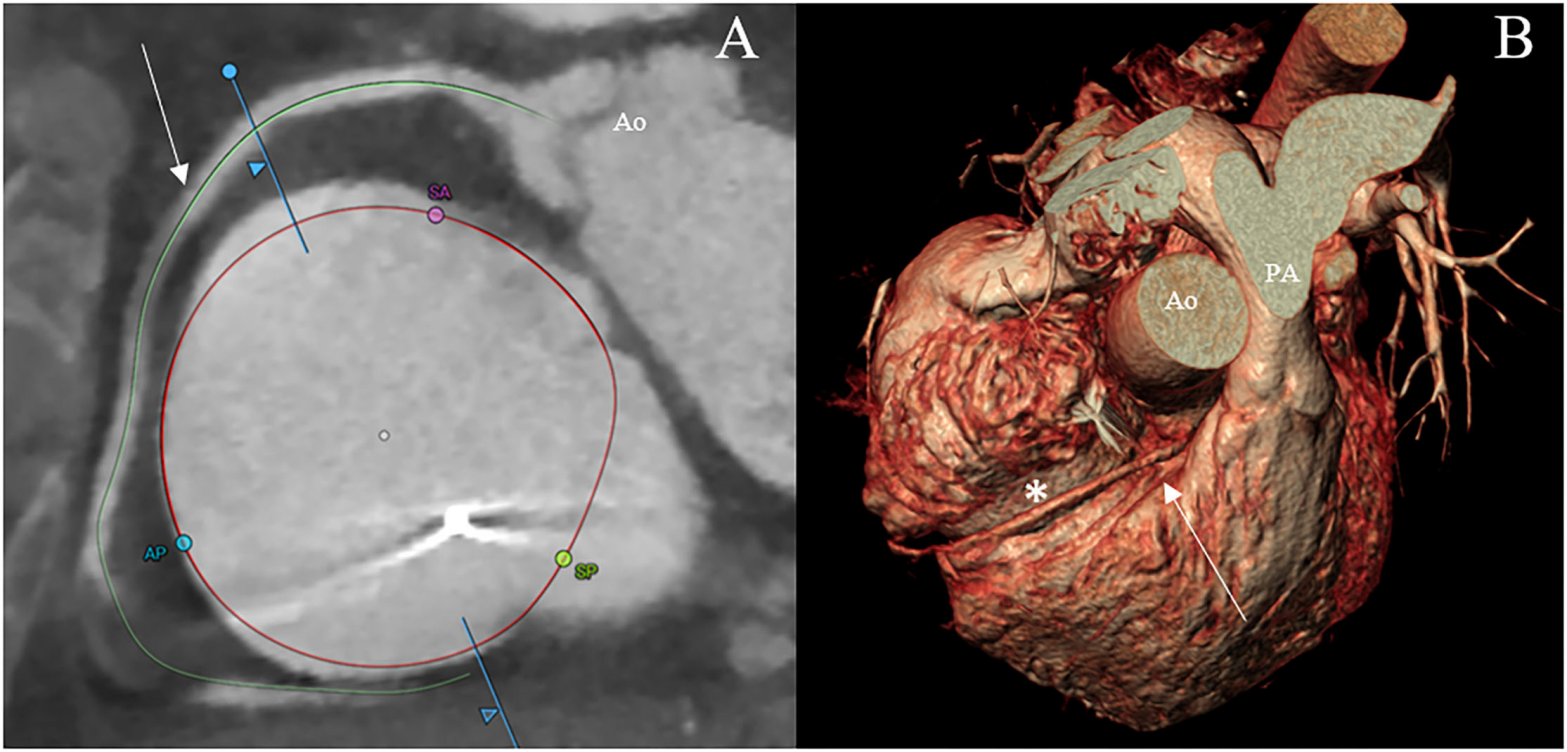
CT scan visualization of the tricuspid annulus (asterisk) and its relationship to the right coronary artery (arrow) using a semiautomated software (3mensio Structural Heart; Pie Medical Imaging, Maastricht, The Netherlands) (A) and the volume renderying technique (B). Ao, aorta; AP, anteroposterior commissure; PA, pulmonary artery; SA, septal-anterior commissure; SP, septal-posterior commissure.
